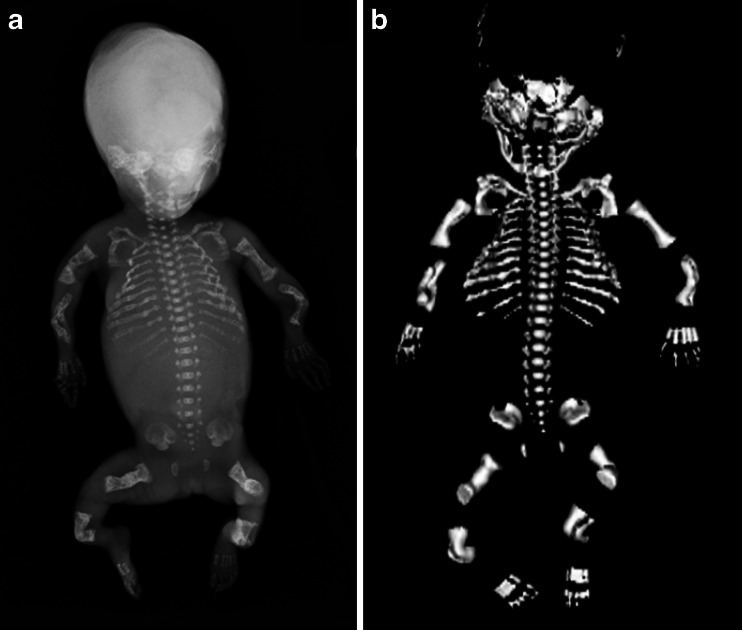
Postmortem skeletal radiograph (a) and 3-D postmortem CT reconstruction (b) of a 20-week male foetus. The pregnancy was terminated for suspected skeletal dysplasia. There is severe under-mineralisation of the entire skeleton, beading of the ribs and bowing and crumpling of the long bones, caused by multiple fractures. These appearances are typical for lethal congenital osteogenesis imperfecta (type II), which was confirmed on genetic testing
